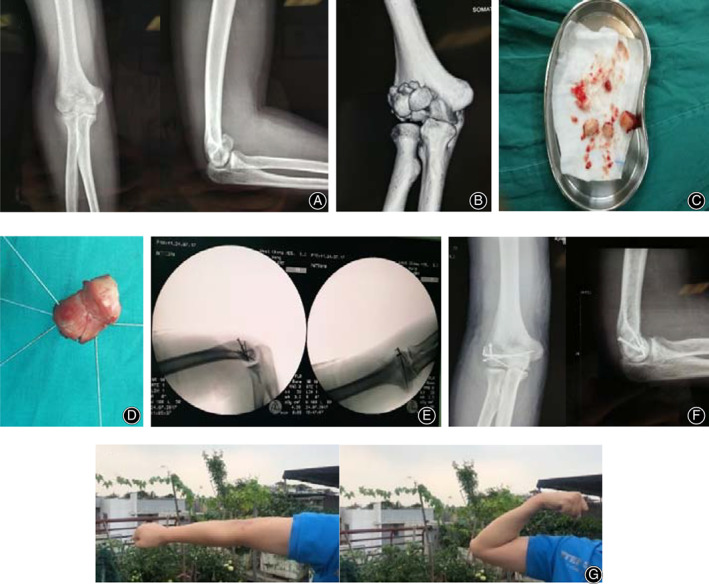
(A) X‐ray films of the fracture. The lateral view showed typical “double arc” sign. (B) Comminuted fracture fragments can be seen in three‐dimensional CT reconstruction. (C) The fracture fragments were taken out of the body. (D) The fragments were fixed as a whole with 1.0mm‐diameter Kirschner wires. (E) X‐ray films after the fracture were fixed with headless screw (DART‐FIRE headless screw produced by WRIGHT company, Memphis, USA) and 1.0mm diameter Kirschner wires. (F) One year after operation, the X‐ray films showed the fracture had healed and the implants were not failure. (G) The flexion and extension images of the elbow 1 year after operation, the MEPS was 90.
